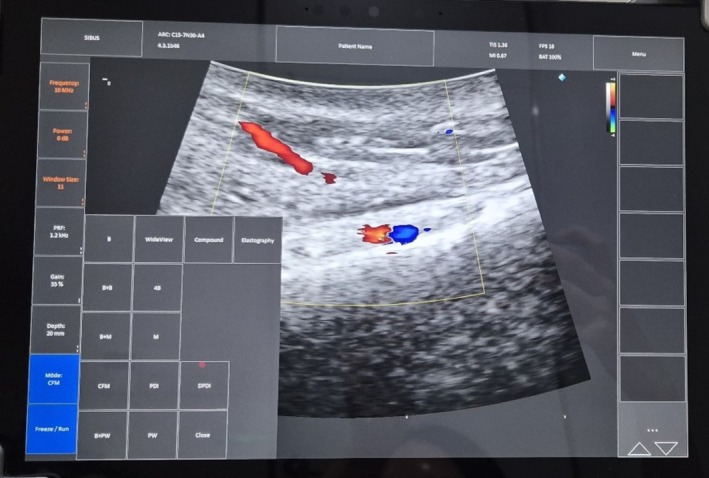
Doppler ultrasound setting. High‐resolution 15 MHz was applied.
